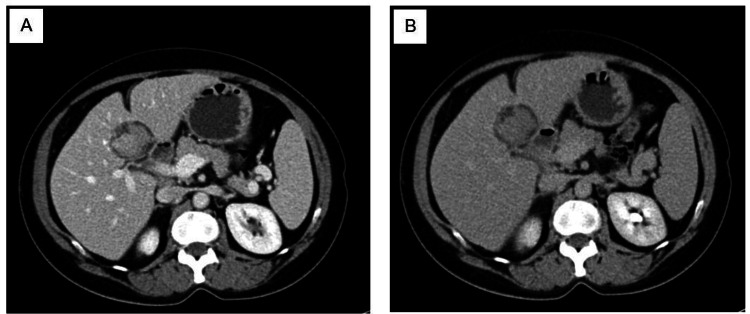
CT findings during arterial (A) and venous phase (B) showed a well-defined focal lesion measuring about 3.3 cm x 3.2 cm x 3.9 cm.
